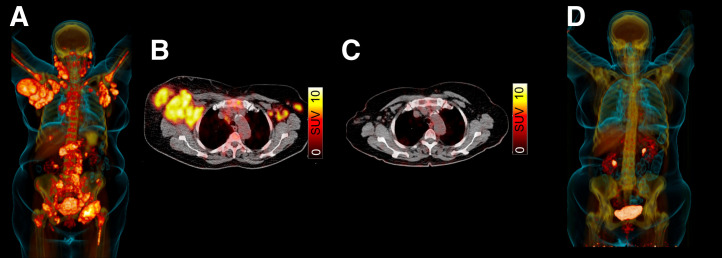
Patient with MZL and scheduled for rituximab-bendamustine. (A and B) Maximum-intensity projection (A) of ^68^Ga-pentixafor PET revealed multiple lymphoma manifestations, in particular in axilla as seen on transaxial PET/CT (B). (C and D) After treatment, complete remission was achieved on follow-up imaging, indicating that CXCR4-targeted PET/CT may be useful to monitor treatment response. (Modified from (53).)
